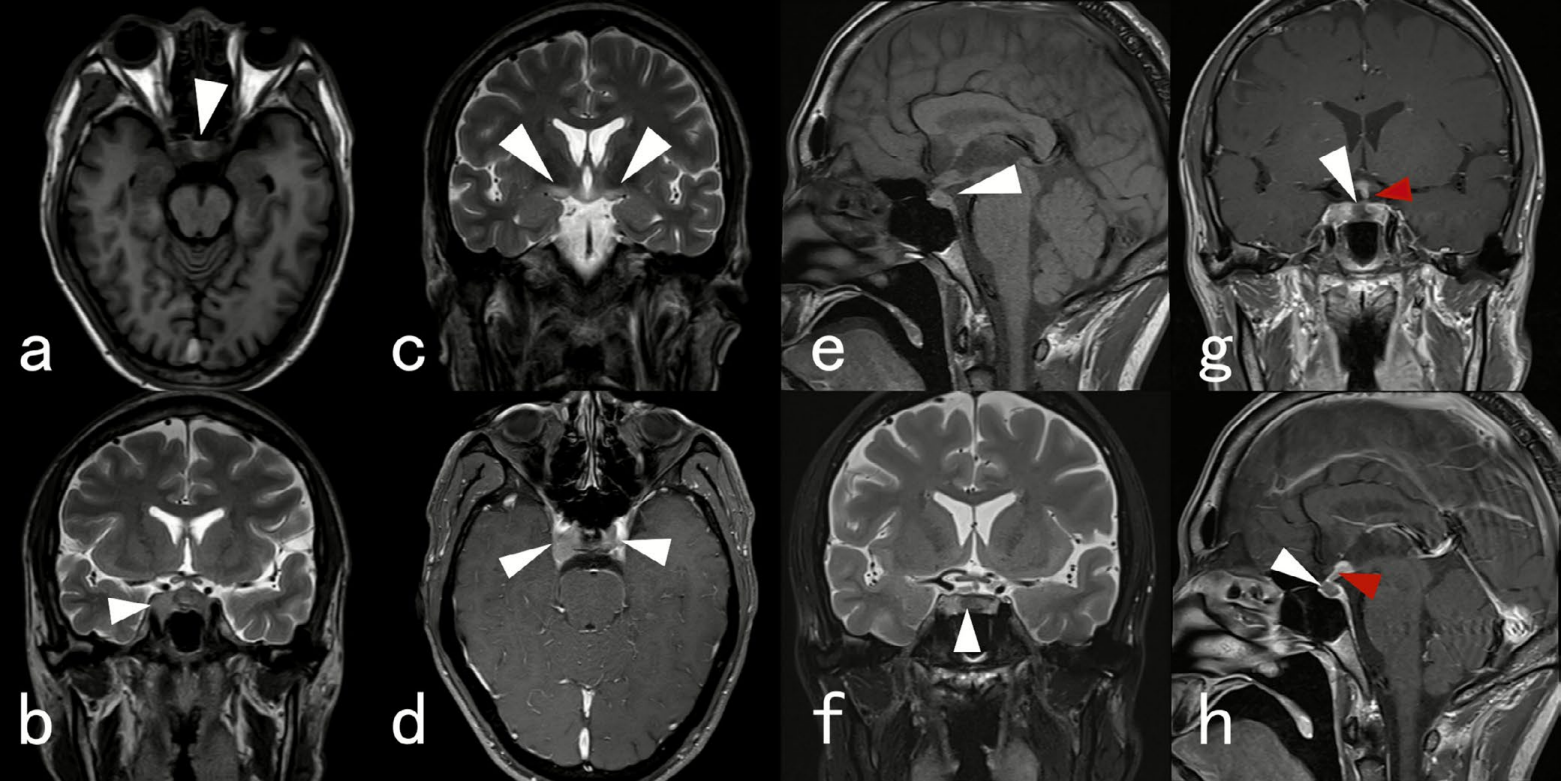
First hospital MRI: (a) high signal intensity on T1‐weighted imaging (T1WI) as indicated by the arrow. (b) Mass‐like T2‐weighted signal (T2WI) lesions visible paranasophally and suprasellar, with bilateral cavernous sinus involvement. (c) Symmetrical edema visible in both thalami. (d) Marked enhancement observed on contrast‐enhanced imaging. Second hospital MRI. (e) Indicates pituitary gland fullness with prominent posterior lobe, upward bulging, height approximately 7.6 mm. T1WI shows no marked hyperintensity in posterior lobe. (e, f) Pituitary signal appears slightly hypointense on T1WI and T2WI. (g, h) White arrows indicate no significant enhancement on contrast‐enhanced scan. Red arrows indicate uneven thickening of the pituitary stalk with localized leftward displacement; the stalk demonstrates markedly heterogeneous enhancement on contrast‐enhanced imaging.
